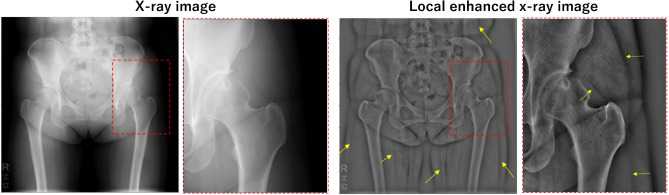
Muscle edges observed in a contrast-enhanced X-ray image. The X-ray image and its contrast-enhanced image^10,11^ are shown on the left and right, respectively. The lower panels show magnified view. The yellow arrows indicate the muscle edges confirmed by an expert surgeon.
